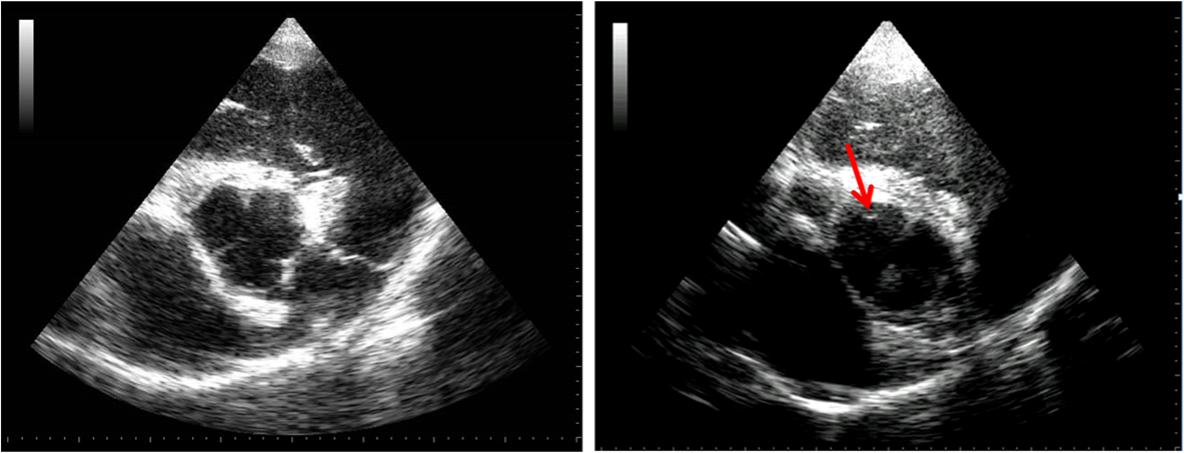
Right parasternal short axis echocardiograms of the aortic valve (AoV). The image on the left shows a view of a normal AoV in the centre of the image as well as the landmarks described in the optimisation study. The image on the right is from aortic valve prolapse (AVP) of the non-coronary cusp (NCC). The images shows the edges of the right and left coronary cusps and the red arrow is highlighting the NCC not being present within the imaging plane as the other two are.
